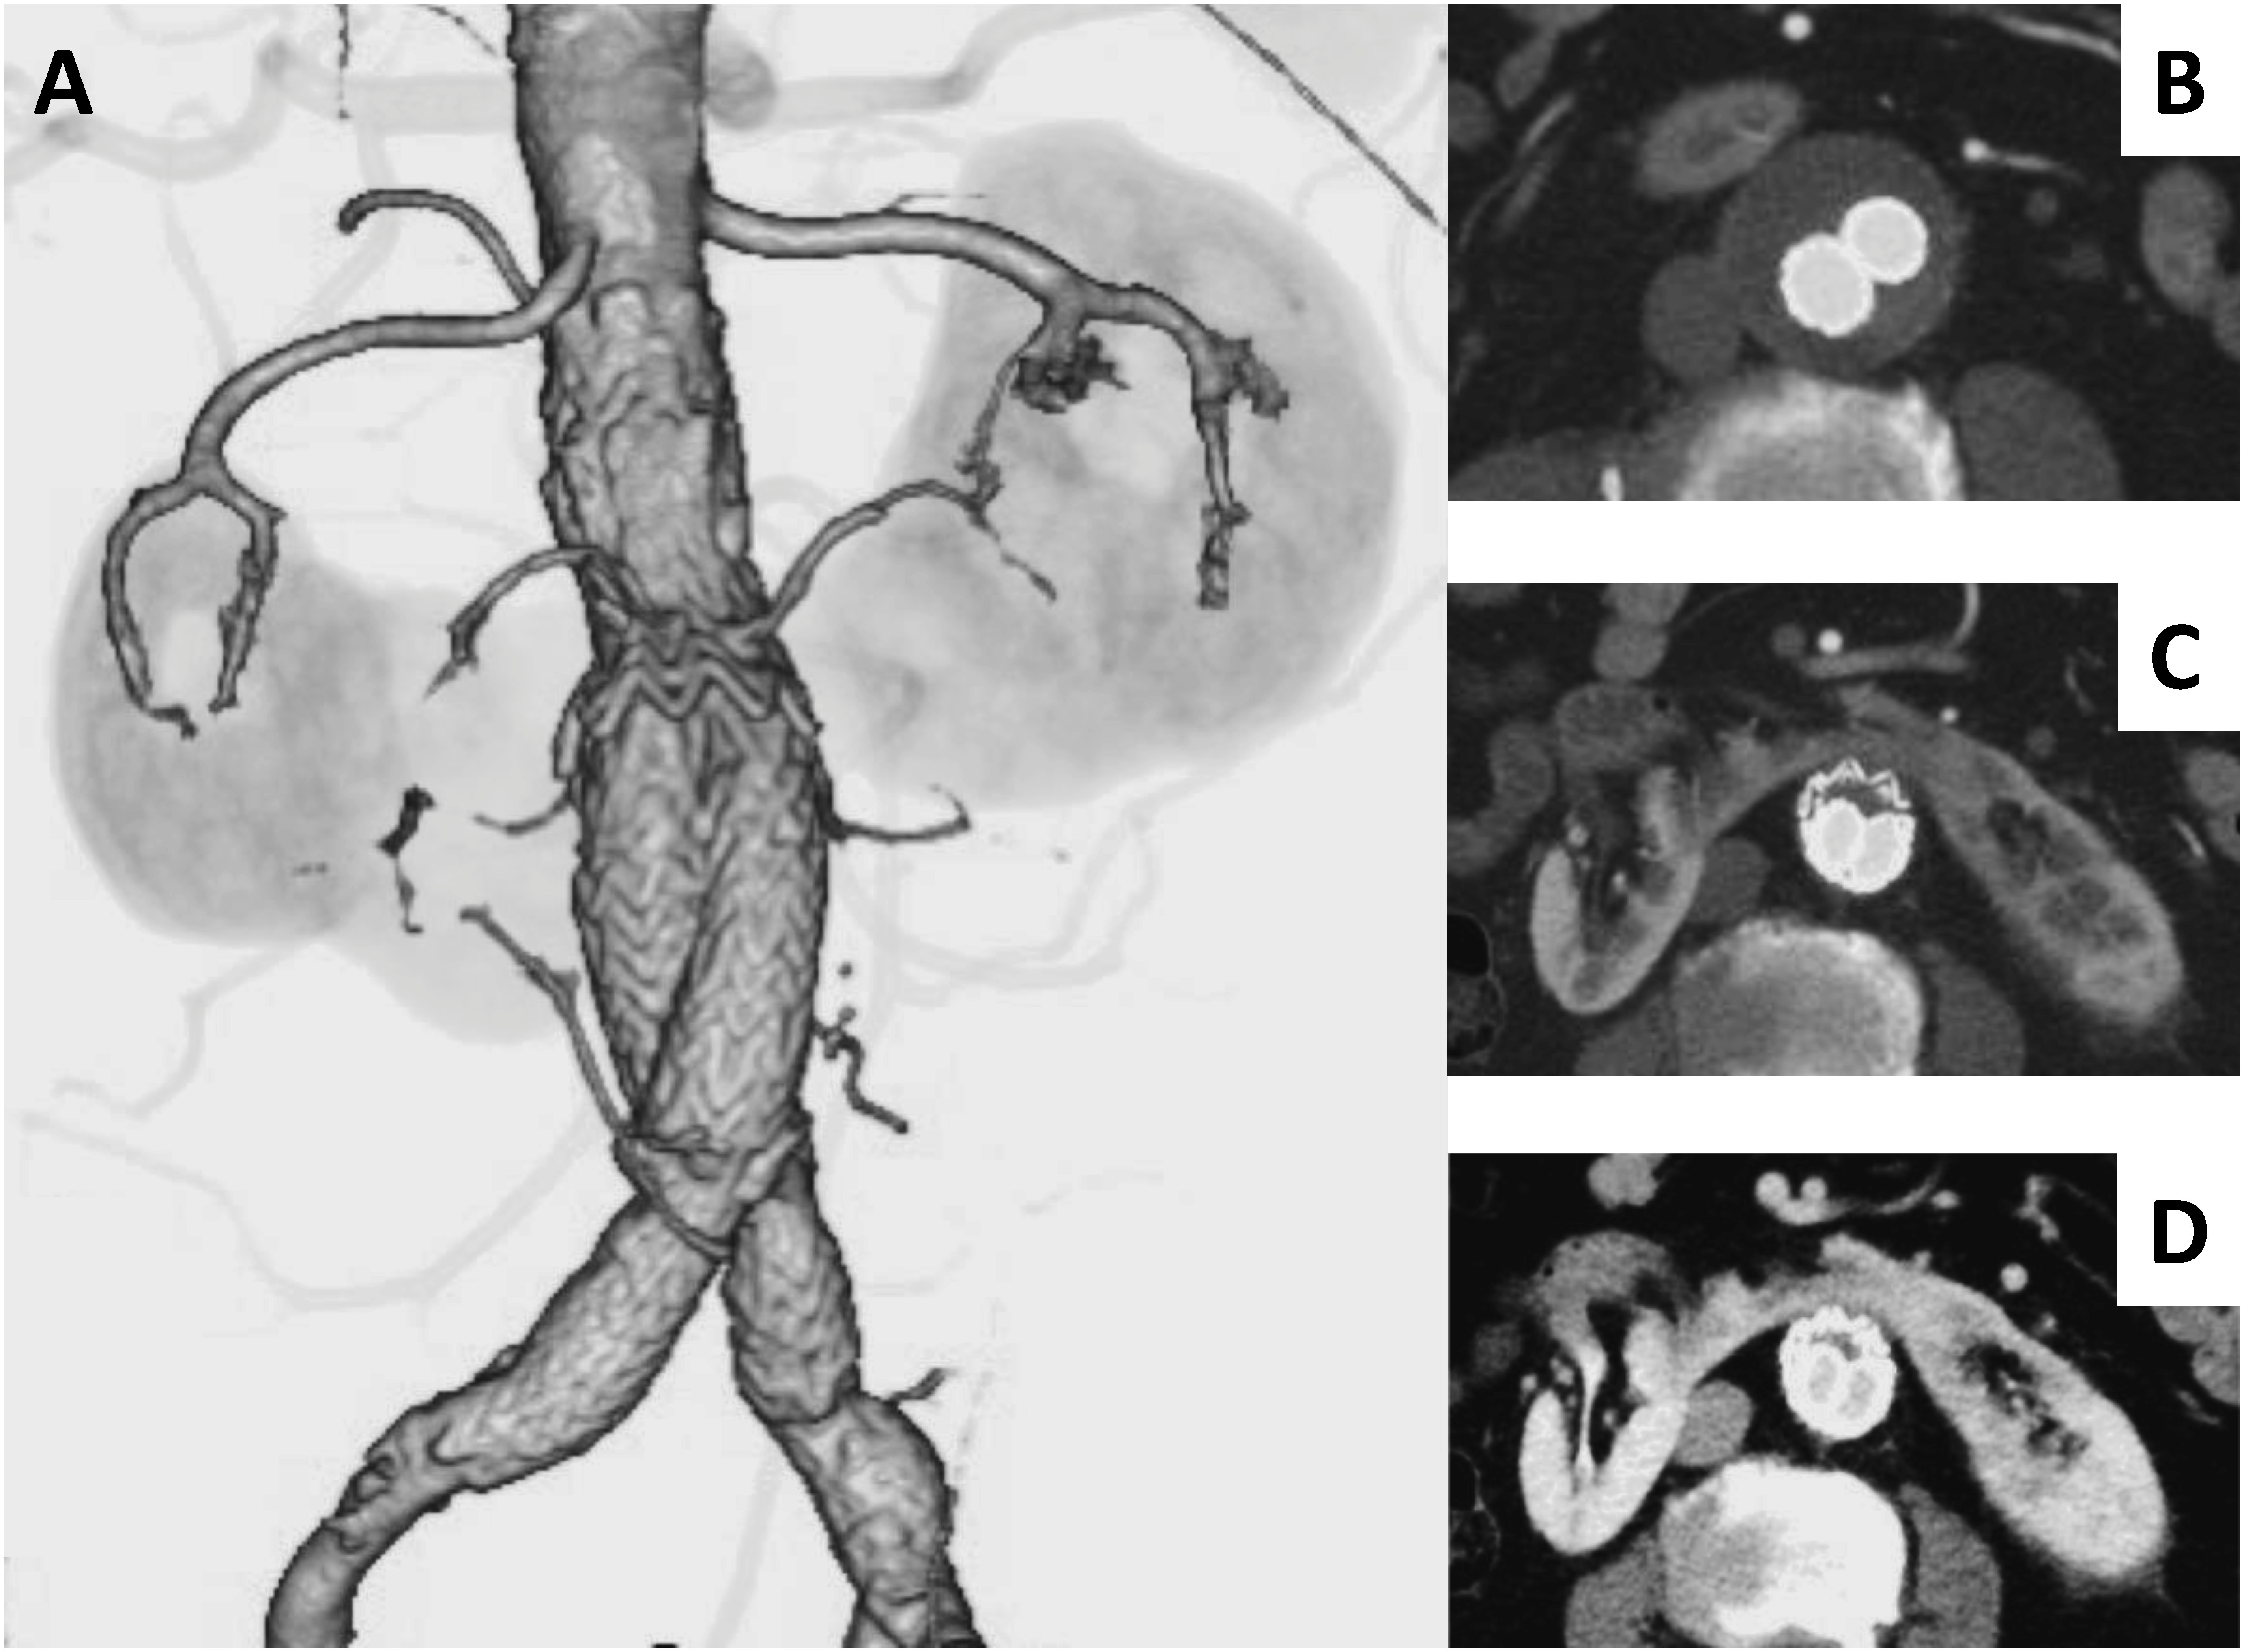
Fig. 3 Postoperative contrast-enhanced computed tomography (CT).
